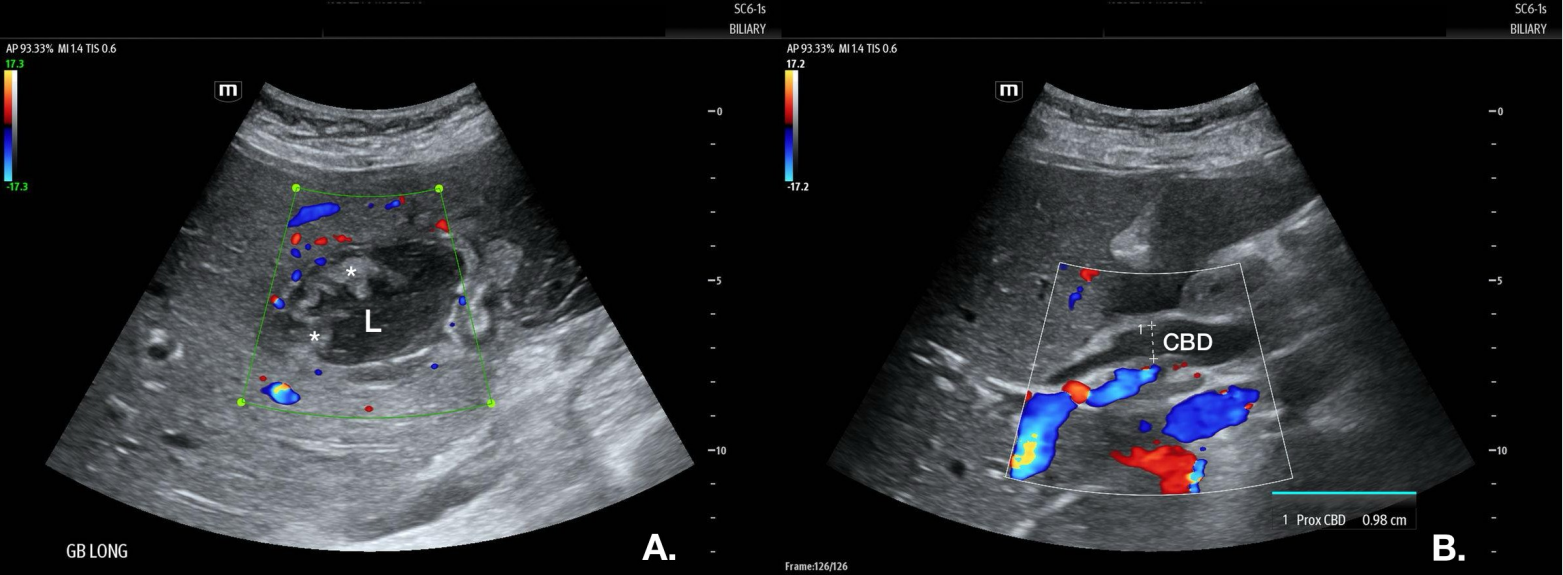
B-mode Ultrasound. B-mode ultrasound (US) with color Doppler overlay of the gallbladder. (Figure 2a) shows hyperechoic echoes (*) in the gallbladder lumen (L) and gallbladder wall thickening with associated hyperemia (red/blue color). The proximal common bile duct is dilated to 0.98 cm (Figure 2b).
